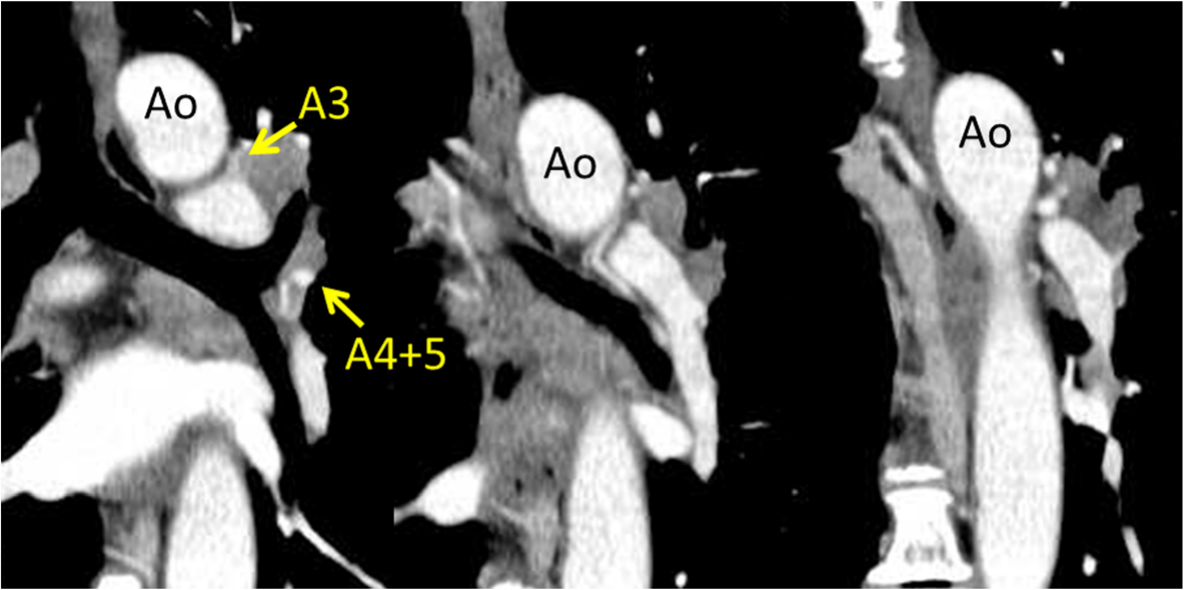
Preoperative computed tomography (serial section of coronal planes). Hilar lymph nodes infiltrating the PA beginning at the first branch to the lingular artery
